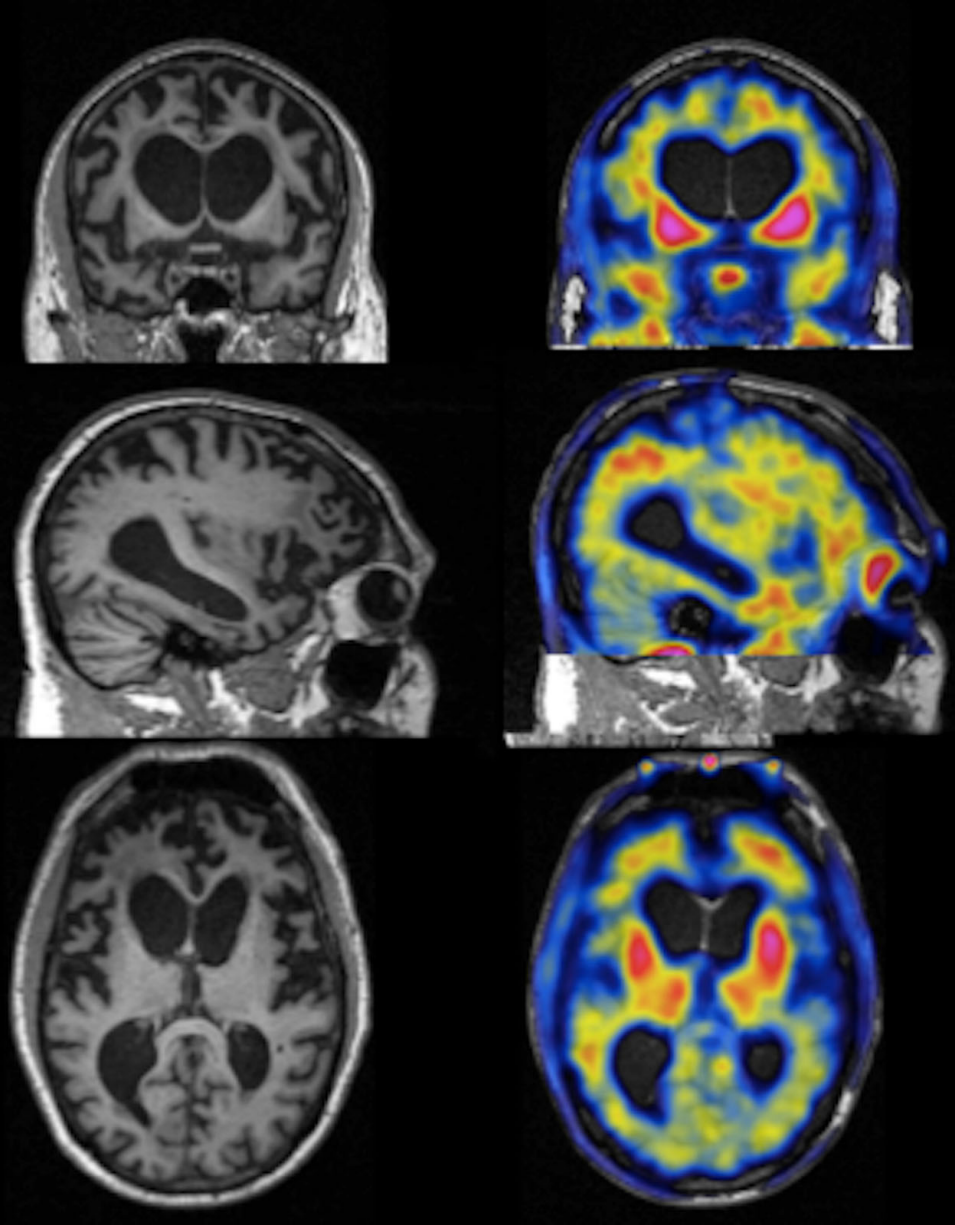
[F18]-T807 PET images from a 56-year-old individual with frontotemporal dementia and the P301L *MAPT* mutation. Coronal (top row), sagittal (middle row) and axial (bottom row) views of prefrontal and anterior temporal atrophy with white matter signal change on MRI (left column) and [F18]-T807 images (right column) showing elevated signal in frontal, anterior temporal and parietal cortex, as well as in basal ganglia, consistent with expected tau inclusions. The PET reference region was the cerebellar grey matter.
